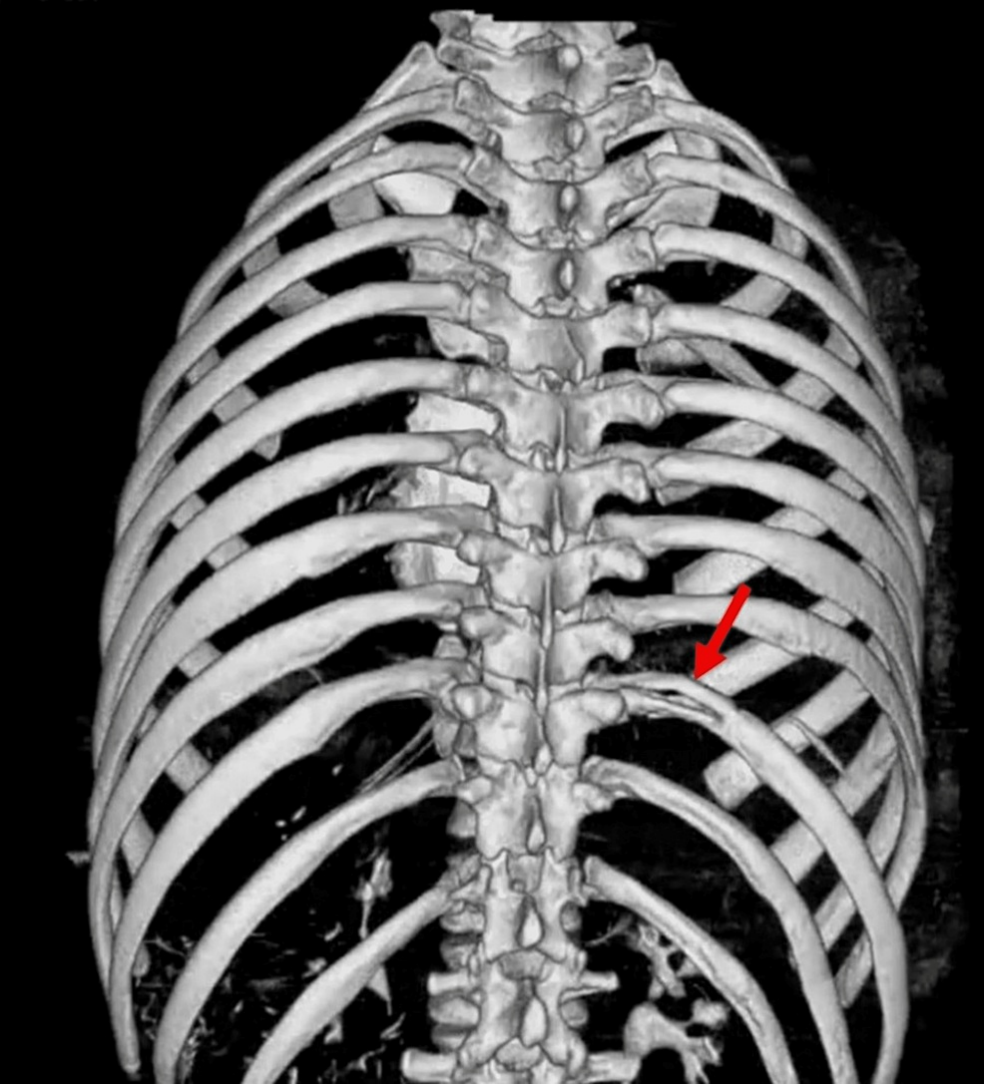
Three-dimensional CT reconstruction showing a posterior misaligned fracture of the right 10th rib (arrow).
